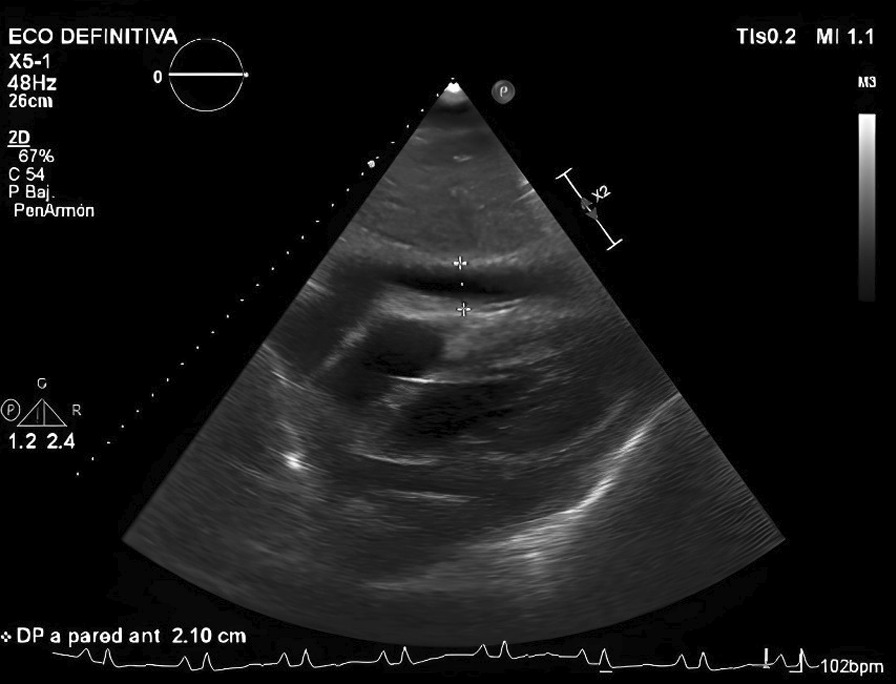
Transthoracic echocardiography showing a massive
